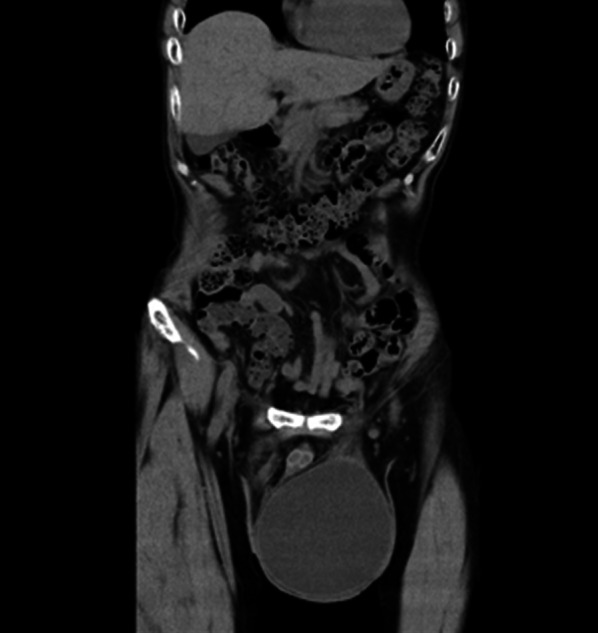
Computed tomography finding of a postoperative giant scrotal fluid collection (hematocele). Pressure on the urethra due to hematocele is observed
